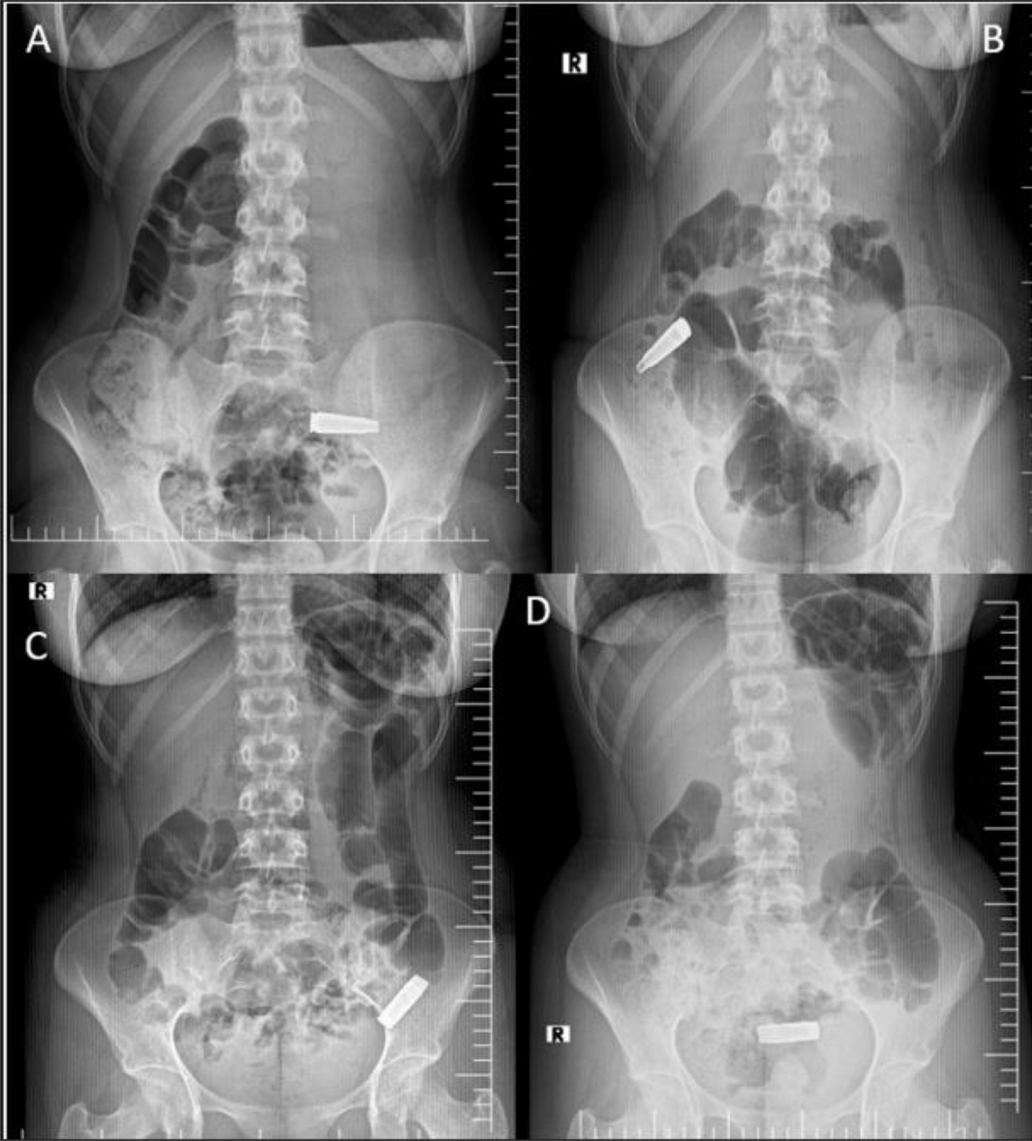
Sequential abdominal radiographs, demonstrated the progressive transit of a bullet shell casing through the gastrointestinal tract, migrating from the terminal ileum (A), to proximal ascending colon (B), to distal descending colon (C) and to the rectum (D)
